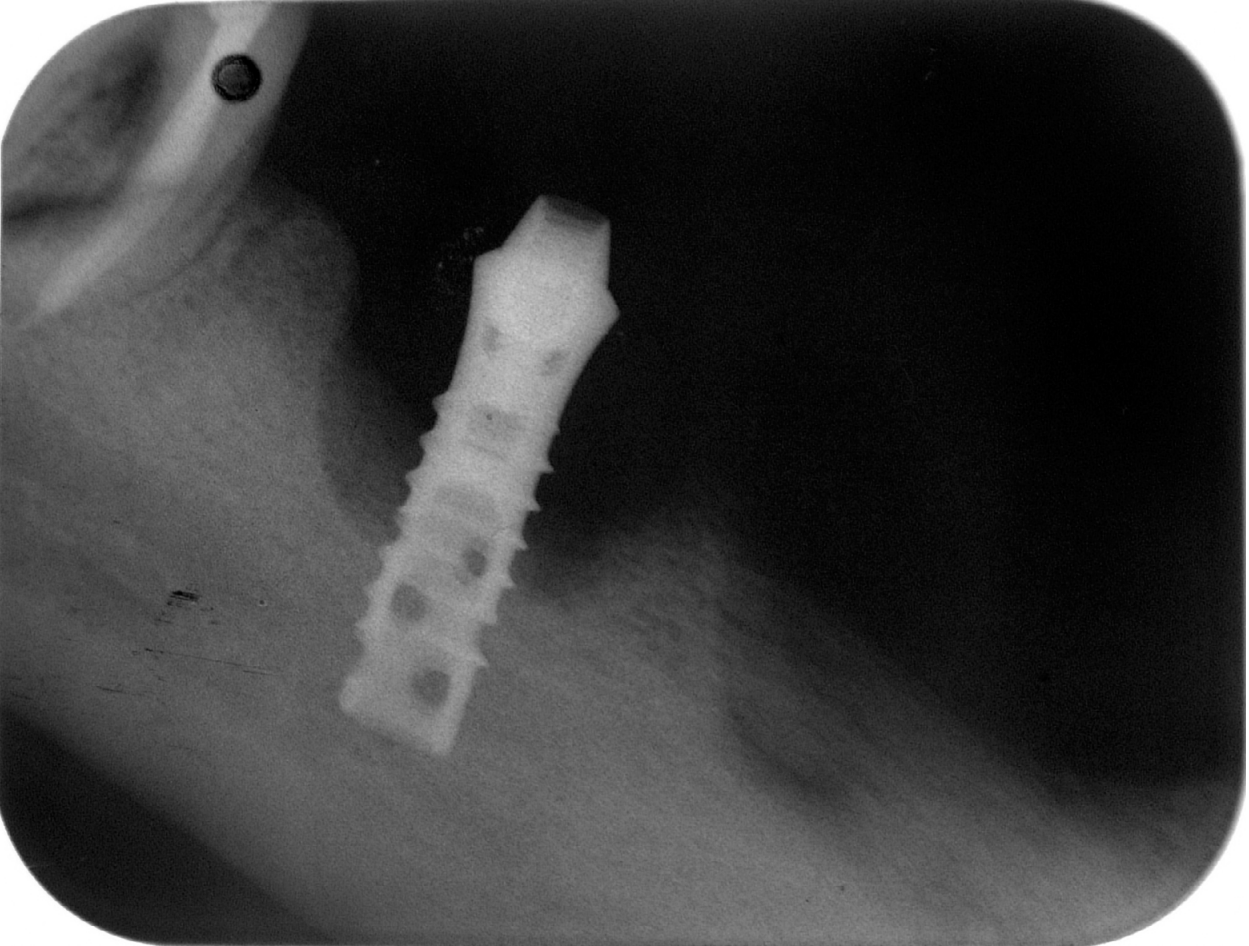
The periapical radiograph of the hollow-screw implant with extensive marginal bone loss.
